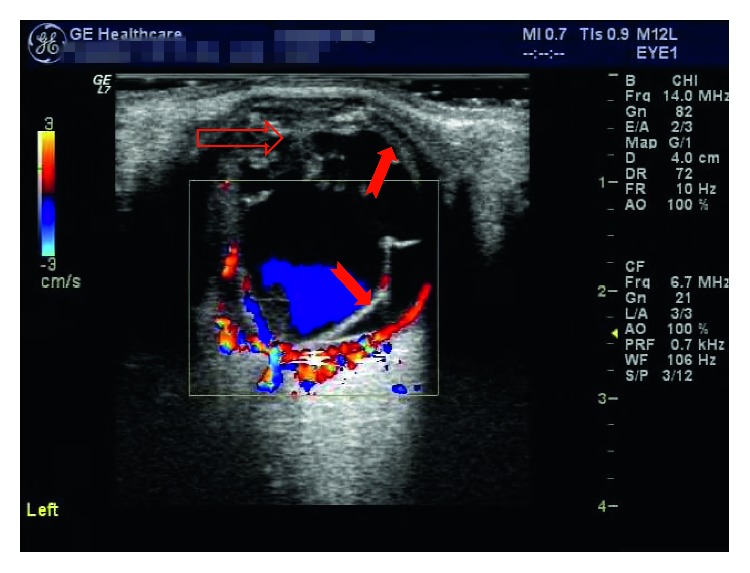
Color Doppler ultrasonography of a patient exhibiting vitreous fibroplasias (indicated by hollow arrows) and choroidal and retinal detachment (indicated by solid arrows) 3 days after repair of the globe.
